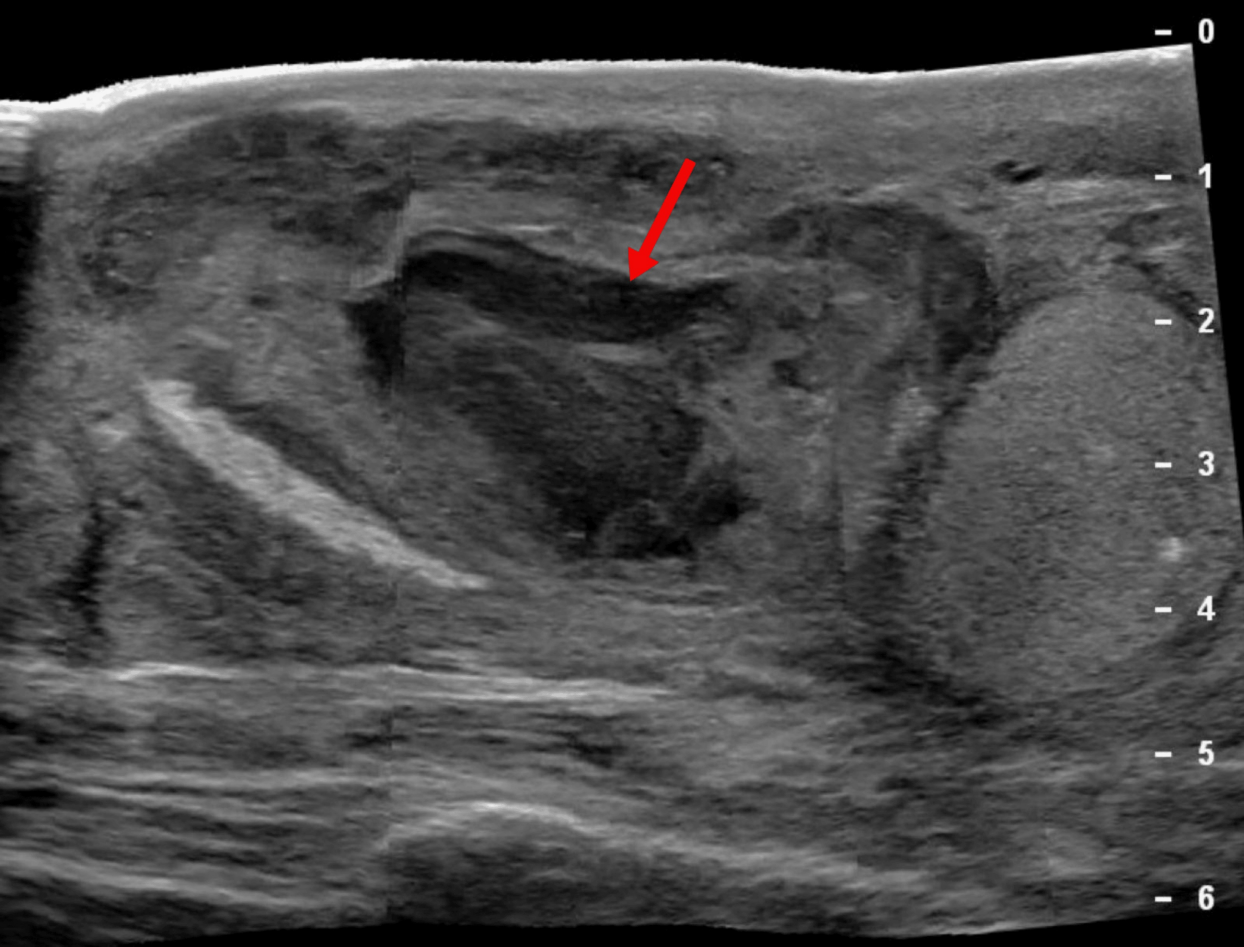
The ultrasound scan reveals findings concerning for a scrotal abscess, showing a right thick-walled extravesicular fluid collection superior to the right testis (arrow).
